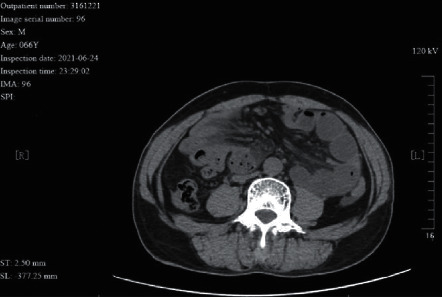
A 66 year-old male patient with paraduodenal hernia. The CT image shows a mesenteric strand sign. No bowel wall ischemia was identified during surgery.
